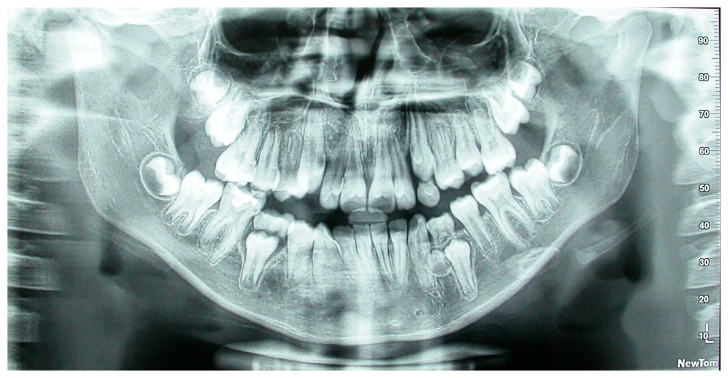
Panoramic radiograph of a patient with supernumerary mandibular left right premolar.
